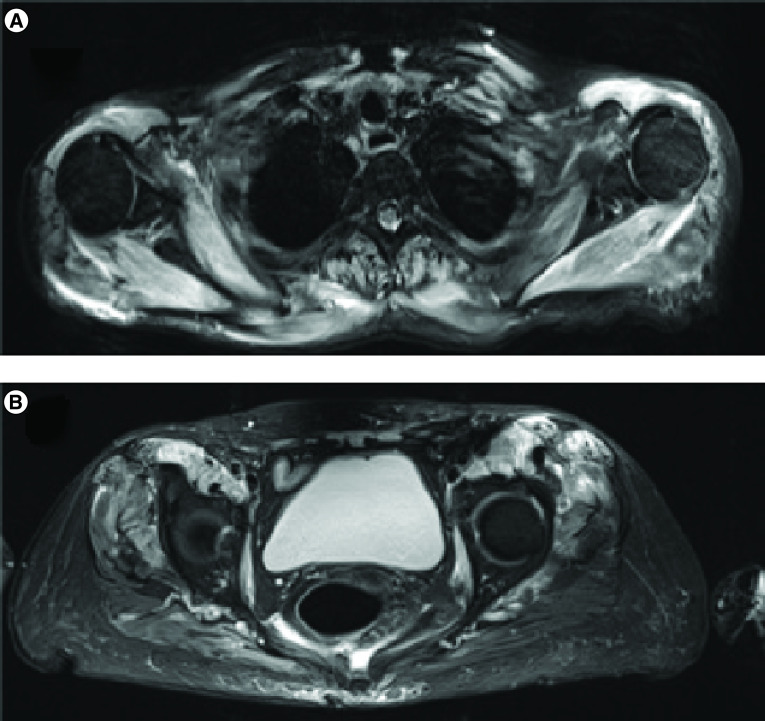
Magnetic resonance imaging showing myositis. Hyperfixation of proximal muscles confirms inflammatory proximal myopathy. (A) Upper limb. (B) Lower limb.
